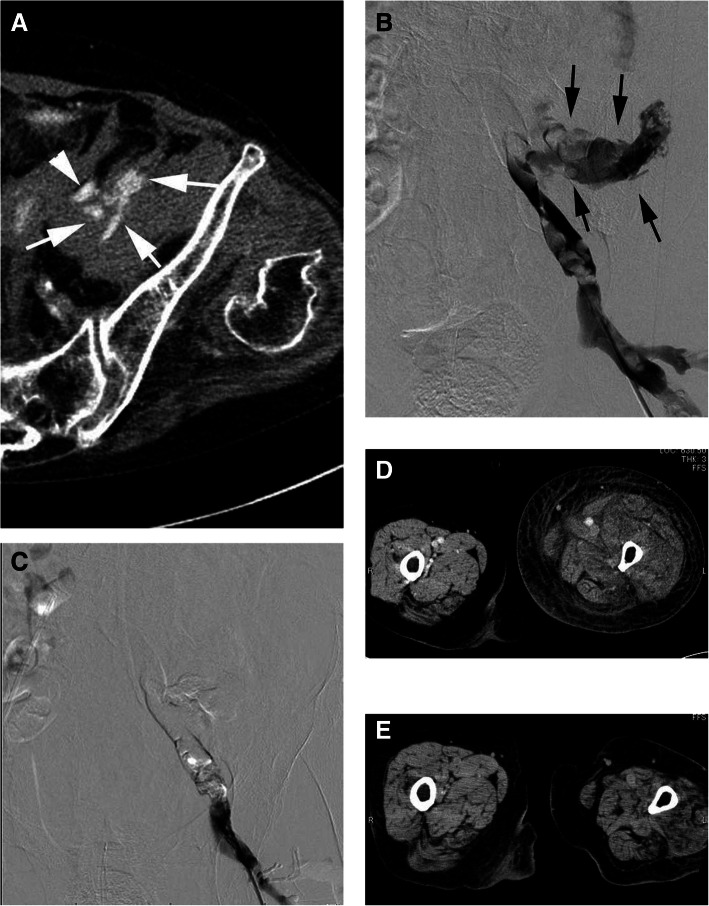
a. Contrast-enhanced CT of the venous phase shows extravasation (arrows) from the lt. external iliac vein (arrowhead). b. DSA shows marked extravasation from the laceration to the adjacent site of the left internal iliac vein (arrows). A thrombus occluded the left common iliac vein. c. DSA shows that the left iliac vein hwas filled and fully embolized with the NBCA/Lipiodol mixture. d. Two days after following embolization, CT shows left leg swelling similar to deep venous thrombosis. e. Six months after embolization, CT shows the resolution of left leg swelling
